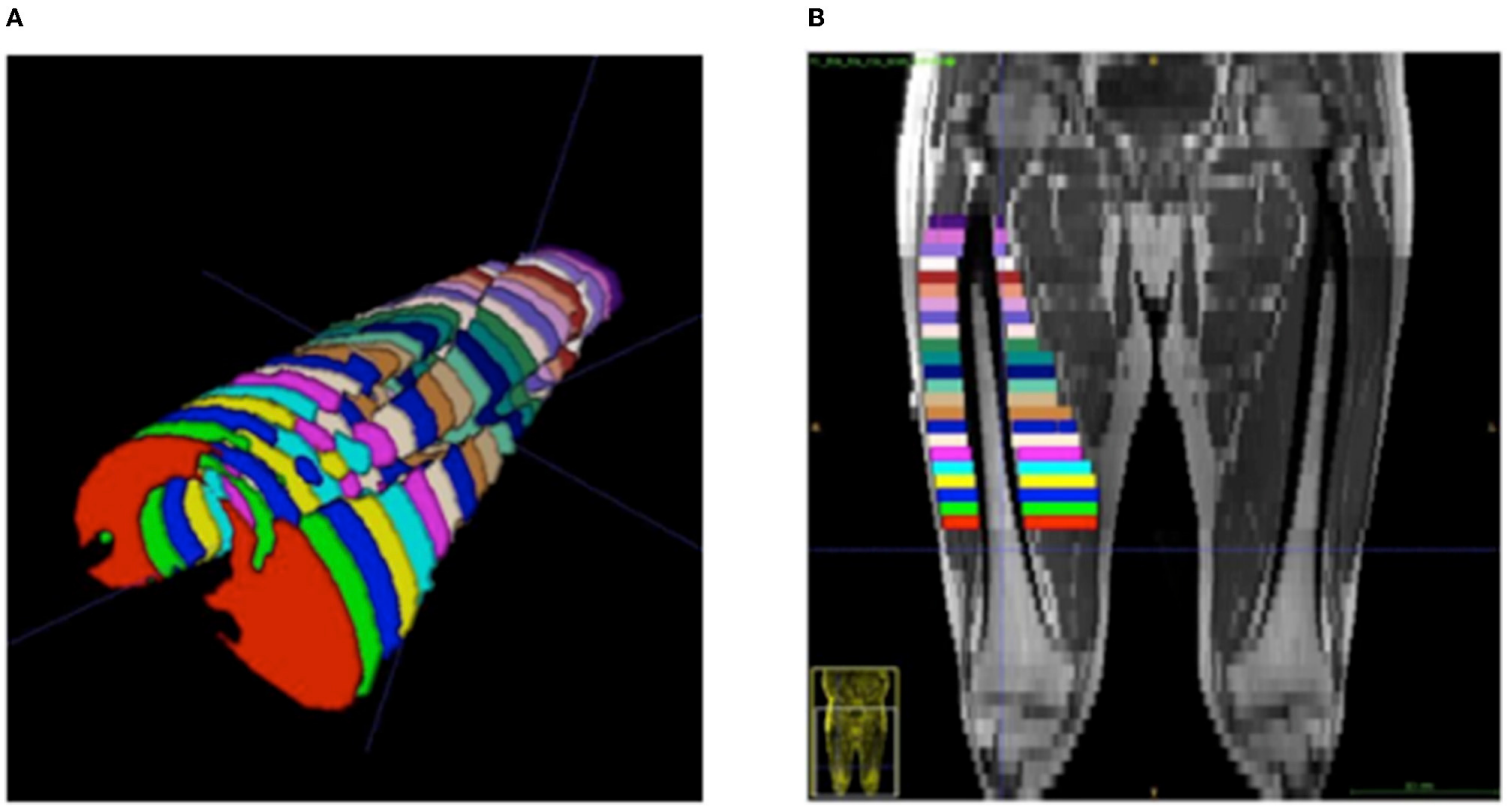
Representative image of a segmented quadricep femoris muscle from an ESLD patient. (A) Viewing in a cephalad direction, each color represents a separate cross section at 1 cm inter-slice distance, (B) a coronal plane MRI demonstrating the distribution and location of equivalent segments.
